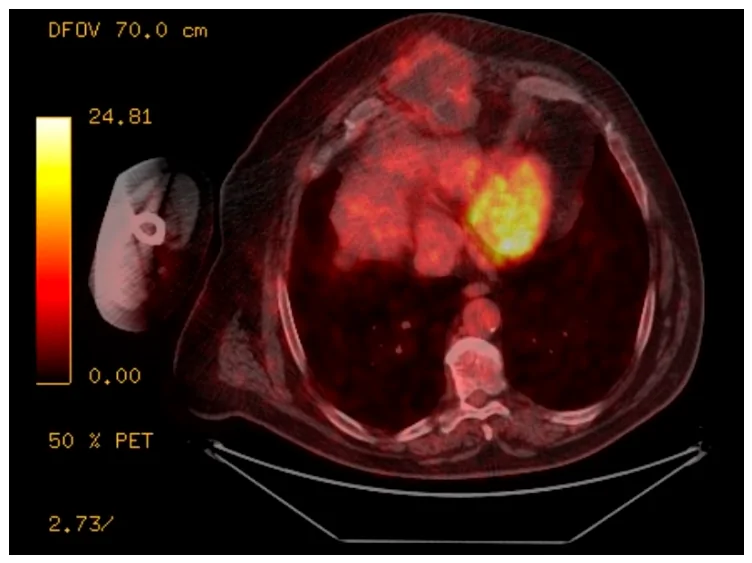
Preoperative axial fused PET/CT caption through the inferior thorax, near the cardiophrenic and diaphragmatic levels.
